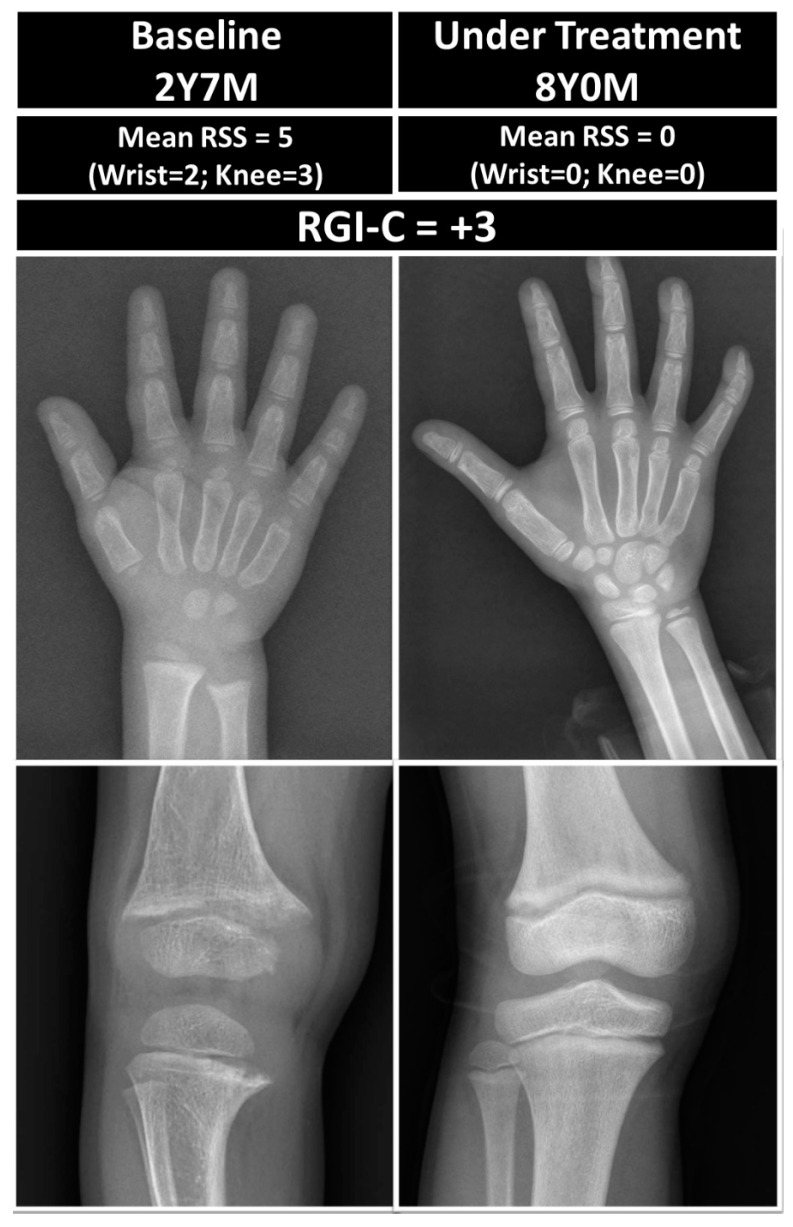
Comparative radiographs and assessment from baseline to 5 years and 6 months post treatment using mean Rickets Severity Score (RSS) and Radiographic Global Impression of Change (RGI-C).
